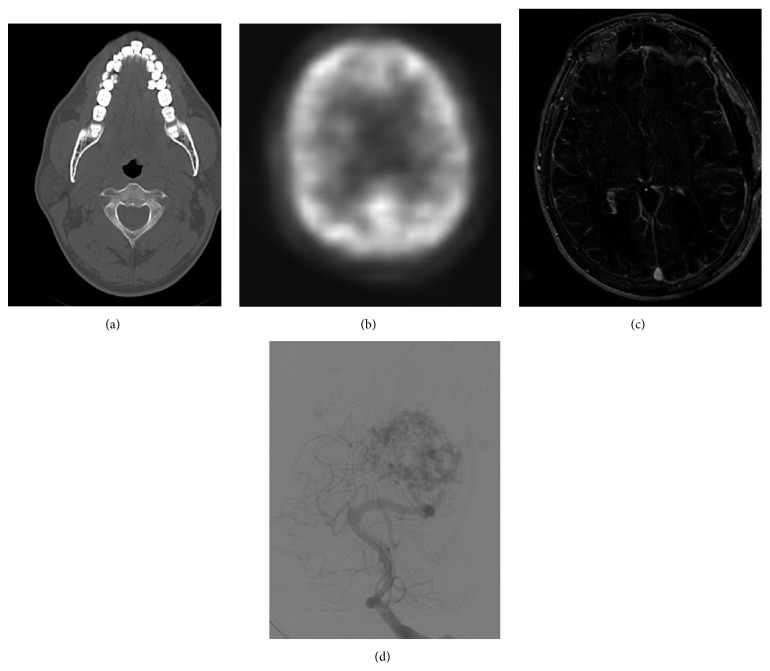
Sample medical images used in the experiment: (a) CT, (b) PET, (c) MR, and (d) XA.
